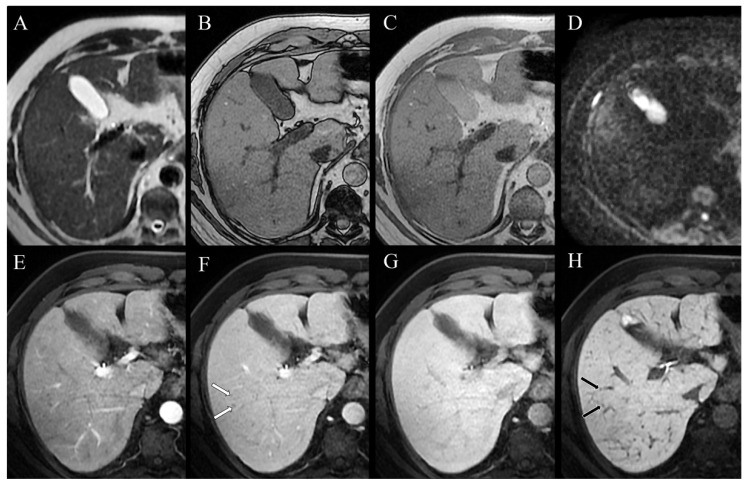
Abdomen magnetic resonance: (A) T2w, (B) T1w out-of-phase, (C) T1w in-phase, (D) DWI, (E) arterial phase, (F) portal venous phase, (G) delayed phase, and (H) hepatobiliary phase images. Millimeter-sized lesions spread throughout the whole liver appear hypointense in all sequences, and do not show enhancement after administration of contrast medium.
